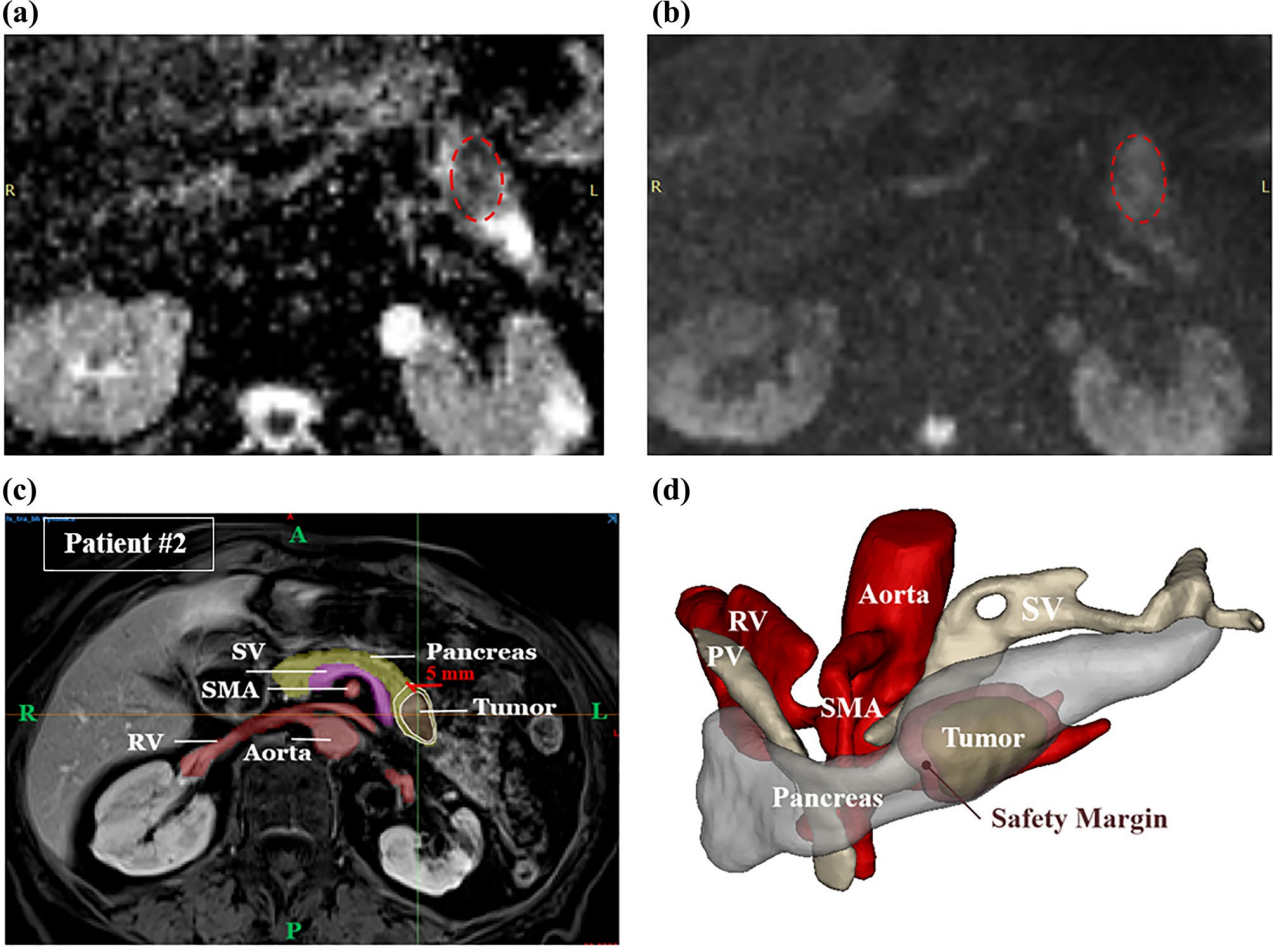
Segmentation of PDAC tumor and adjacent major vessels in abdominal MRI of patient #2; (a) apparent diffusion coefficient map, (b) diffusion-weighted EPI sequence: the red dashed lines indicate the tumor, (c) marked T1-weighted fl3d image: in the body of the pancreas, a solid tumor with an oval morphology and inhomogeneous enhancement caused abrupt interruption of the main pancreatic duct, which shows irregular dilatation downstream along the body-tail, (d) reconstructed anatomical geometry. The segmented T1 image was generated using Materialise Mimics® version 21.0, while the anatomical geometry was created using Materialise 3-matic® version 13.0 software (Materialise, Leuven, Belgium, https://www.materialise.com/en). *PV* portal vein, *SV* splenic vein, *SMA* superior mesenteric artery, *RV* renal vein.
